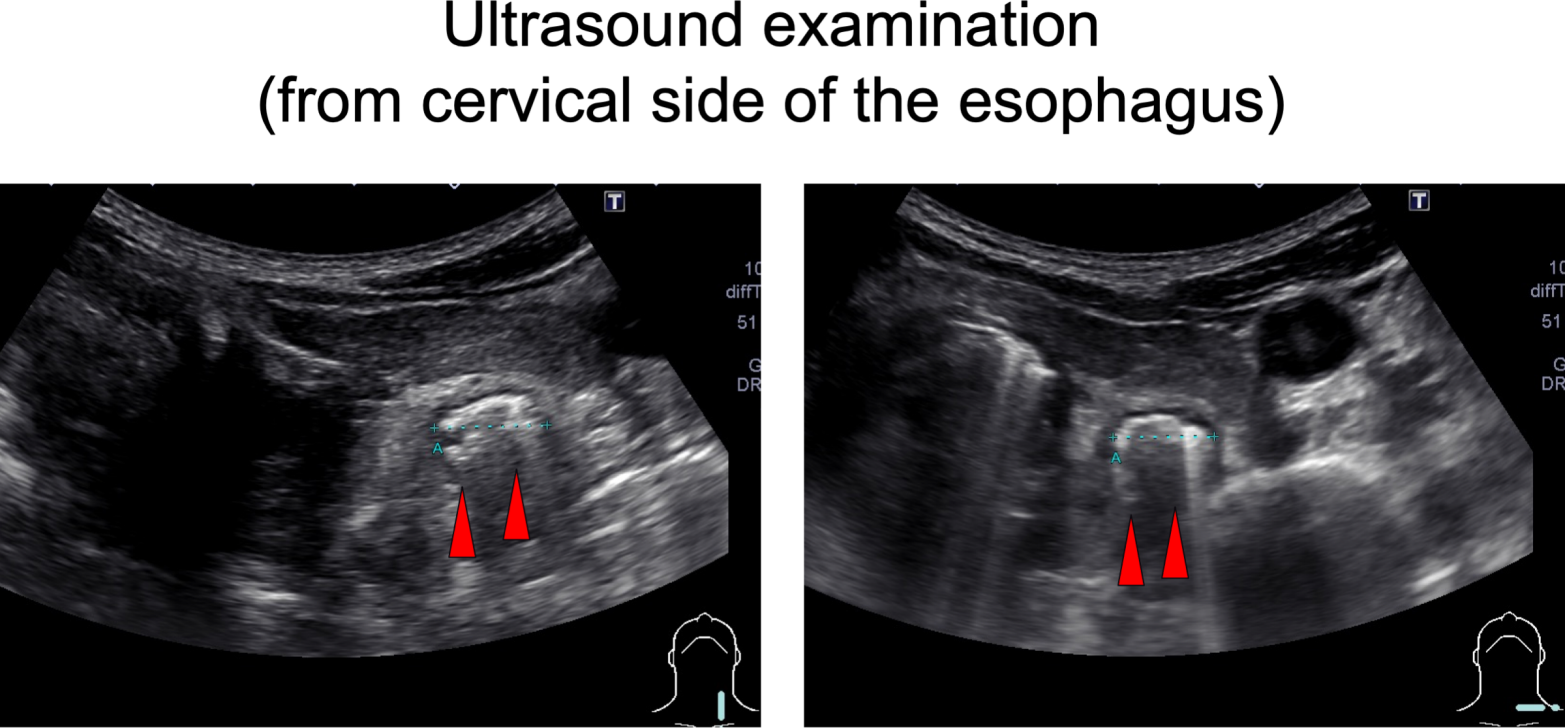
Ultrasound examination. An approximately 1 cm long, high echoic mass was detected in the cervical side of the esophagus (a red arrowhead).
